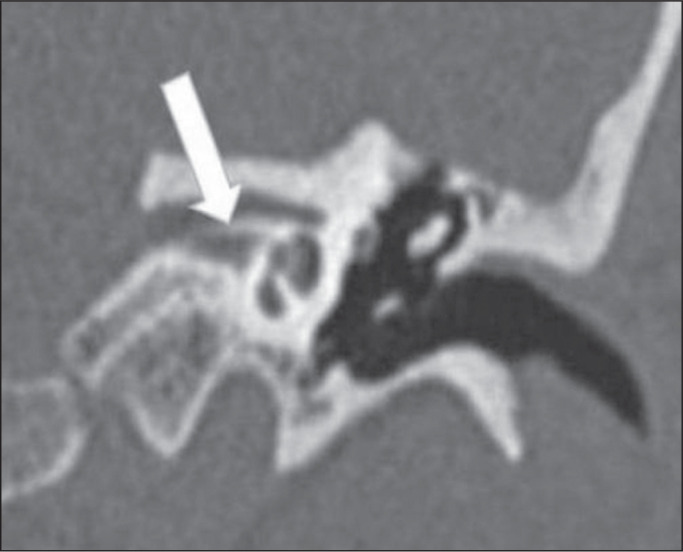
Transverse crest. Unenhanced coronal CT of the temporal bone, showing
